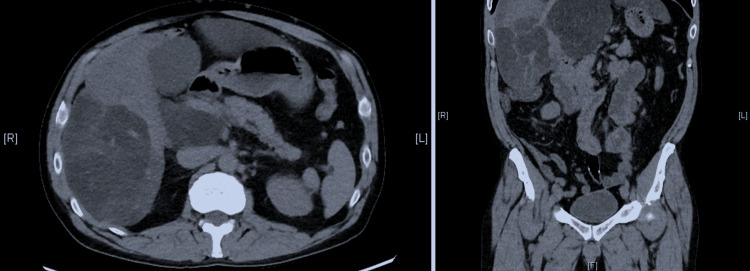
Axial (left panel) and sagittal (right panel) views of the liver on a non-contrast abdominal computed tomography scan, demonstrating hypoattenuated patches in the liver, the "geographic liver" pattern.
